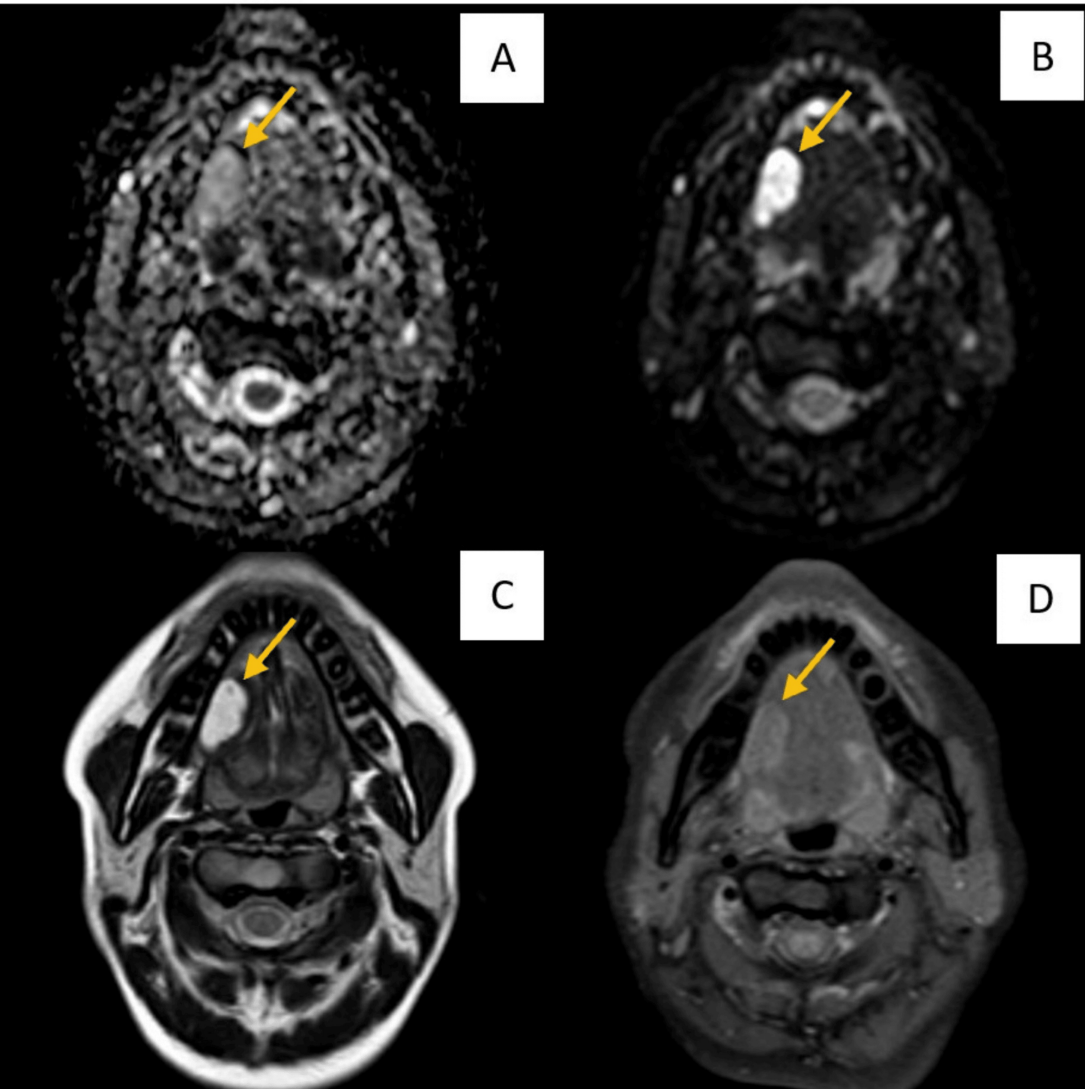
MRI images of an 18-year-old female showing mildly enhancing, fairly defined, diffusion restricting, T2 hyper-intense lesion with internal hypointensity (calcification) in the right anterior lateral aspect of the tongue involving the intrinsic and extrinsic muscles. The view of the central calcific component lesion favors hemangioma A and B: ADC and DWI showing diffusion restriction; C: T2-weighted imaging MRI sequence showing a fairly defined, T2 hyper-intense lesion with an internal hypo-intense focus is seen in the right lateral aspect of anterior 2/3rd of the tongue; D: T1-weighted imaging MRI sequence showing a fairly defined, T2 iso-intense lesion seen in the right lateral aspect of anterior 2/3rd of the tongue
